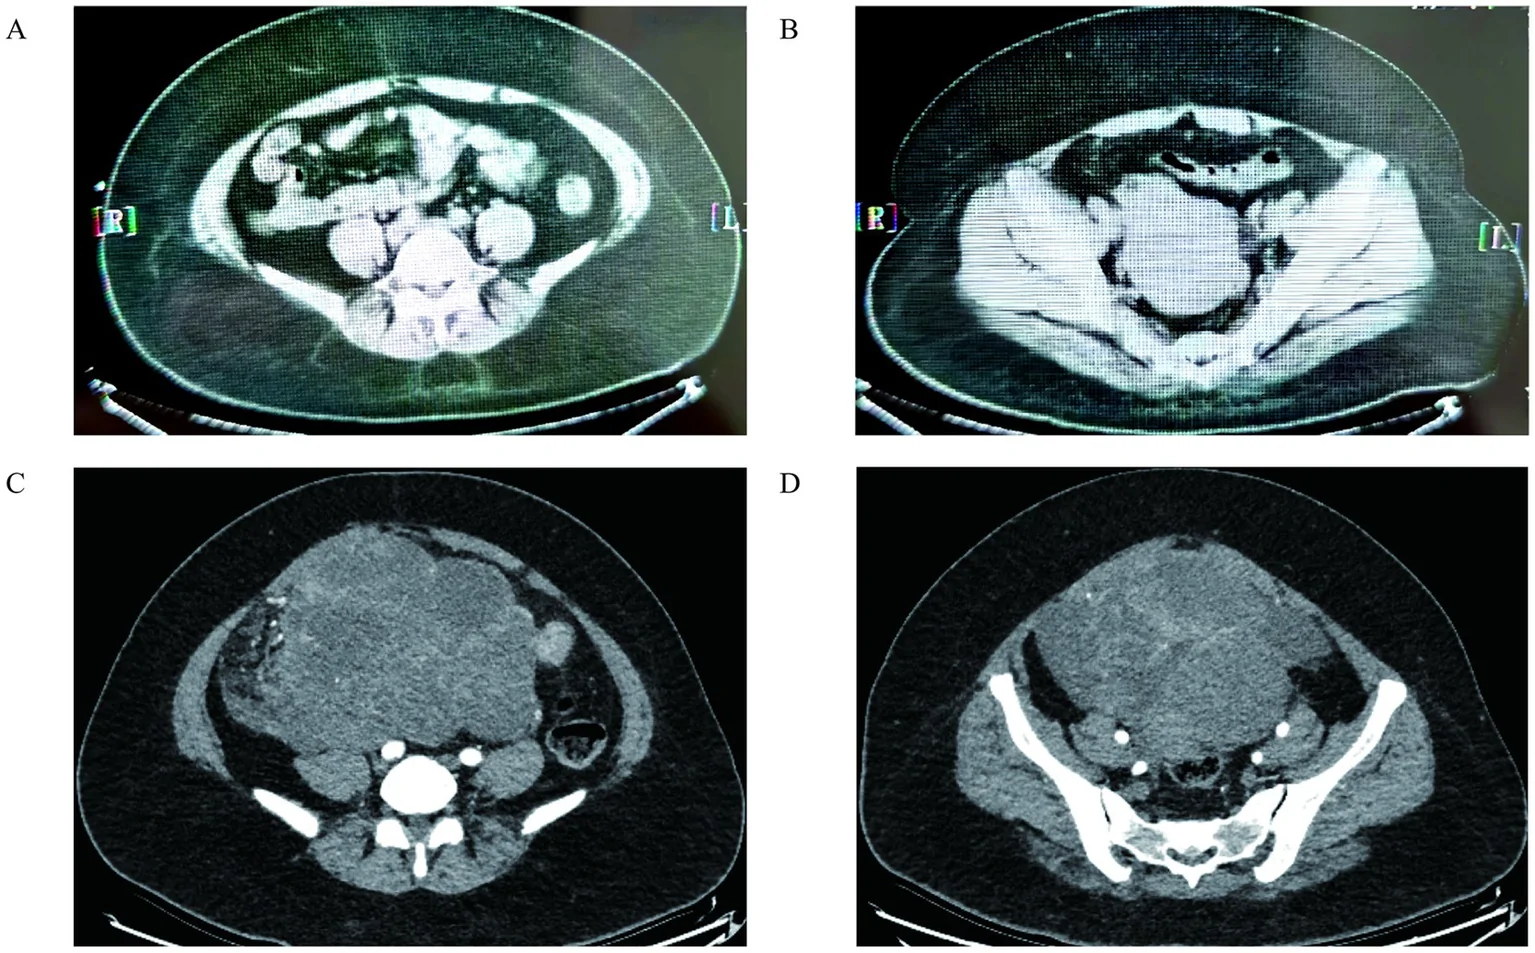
Comparison of abdominopelvic computed tomography (CT) scans between 8 months ago and admission, revealing a hyper-progressive pelvic giant metastasis (A,B) Axial abdominopelvic CT scans performed at a local hospital 8 months ago, showing no obvious organic or space-occupying lesions within the abdominal and pelvic cavities, serving as baseline normal imaging. (C,D) Axial contrast-enhanced pelvic CT scans obtained upon the current admission, demonstrating a massive, mixed density, mixed solid-cystic cystic-solid neoplastic lesion in the lower abdomen and pelvic cavity, measuring approximately 15.2 cm × 13.3 cm × 13.1 cm. The giant pelvic mass is closely adjacent to the adjacent bowel loops and the left adnexa, mimicking a primary gynecological/ovarian tumor.
